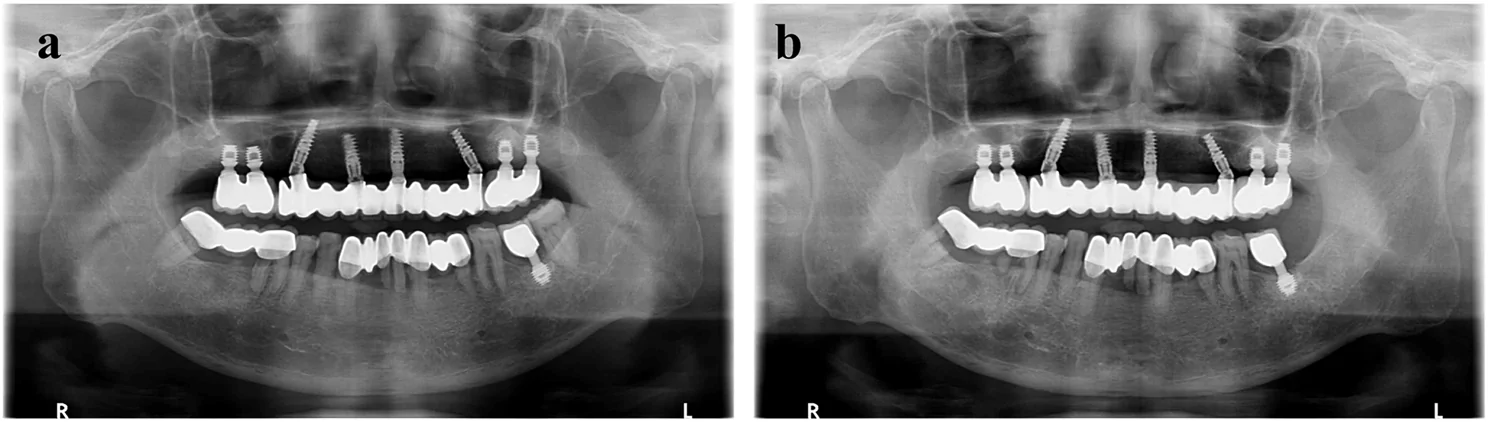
a Panoramic radiograph at the time of definitive restoration delivery. b Follow-up panoramic radiograph at 55 months
